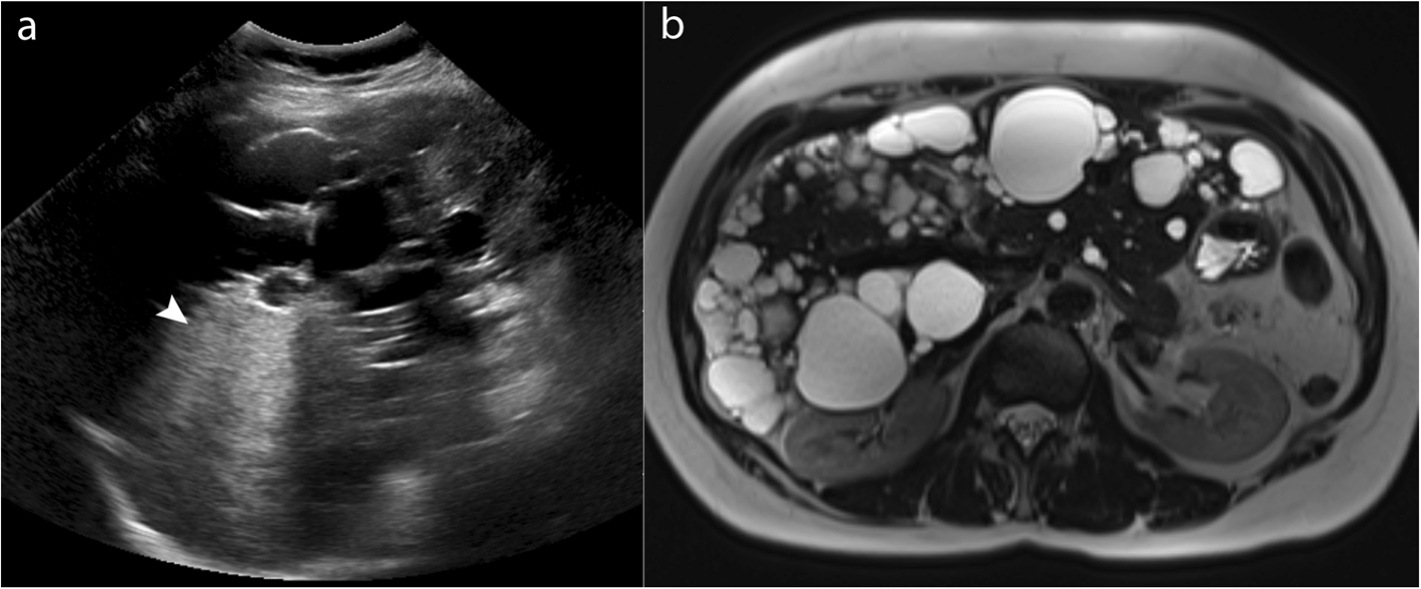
A 58-year-old female patient with chronic hepatitis B and polycystic liver. a Heterogeneous posterior acoustic enhancement (arrowhead) limits lesion detection on ultrasound. b The cysts do not affect liver parenchymal visibility with MRI
